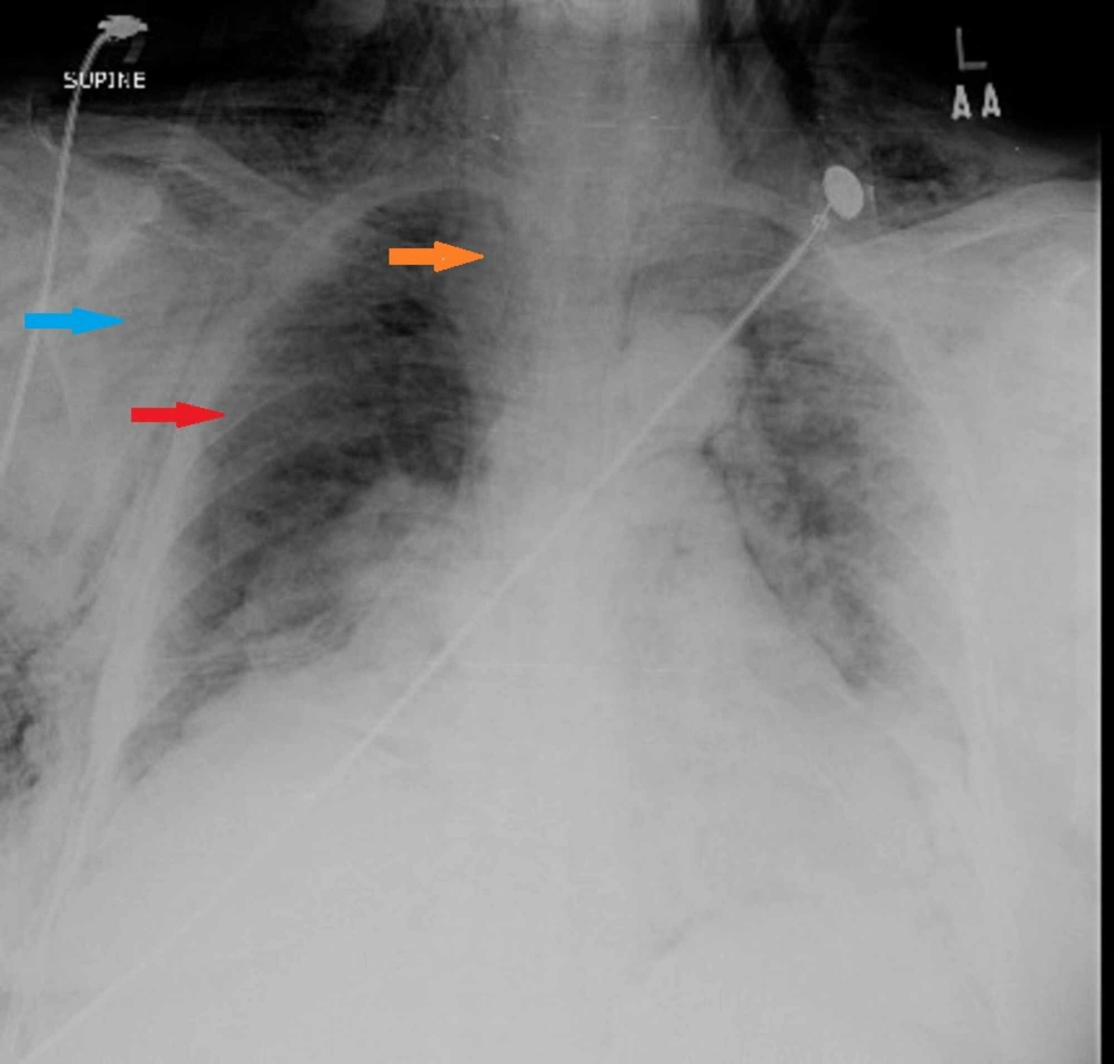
Chest X-ray showing bilateral subcutaneous emphysema (blue arrow), pneumomediastinum (orange arrow), and right-sided pneumothorax (red arrow).
